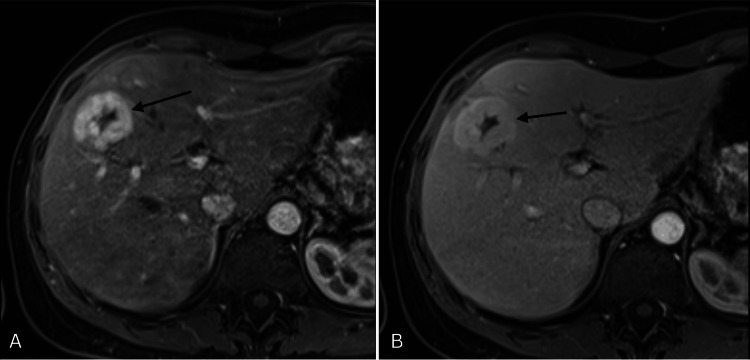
Contrast-enhanced MRI of the liver, black arrows pointing at FNH. (A) Arterial phase: intense enhancement of the lesion. (B) Venous phase: attenuation of contrast uptake MRI: magnetic resonance imaging, FNH: focal nodular hyperplasia
